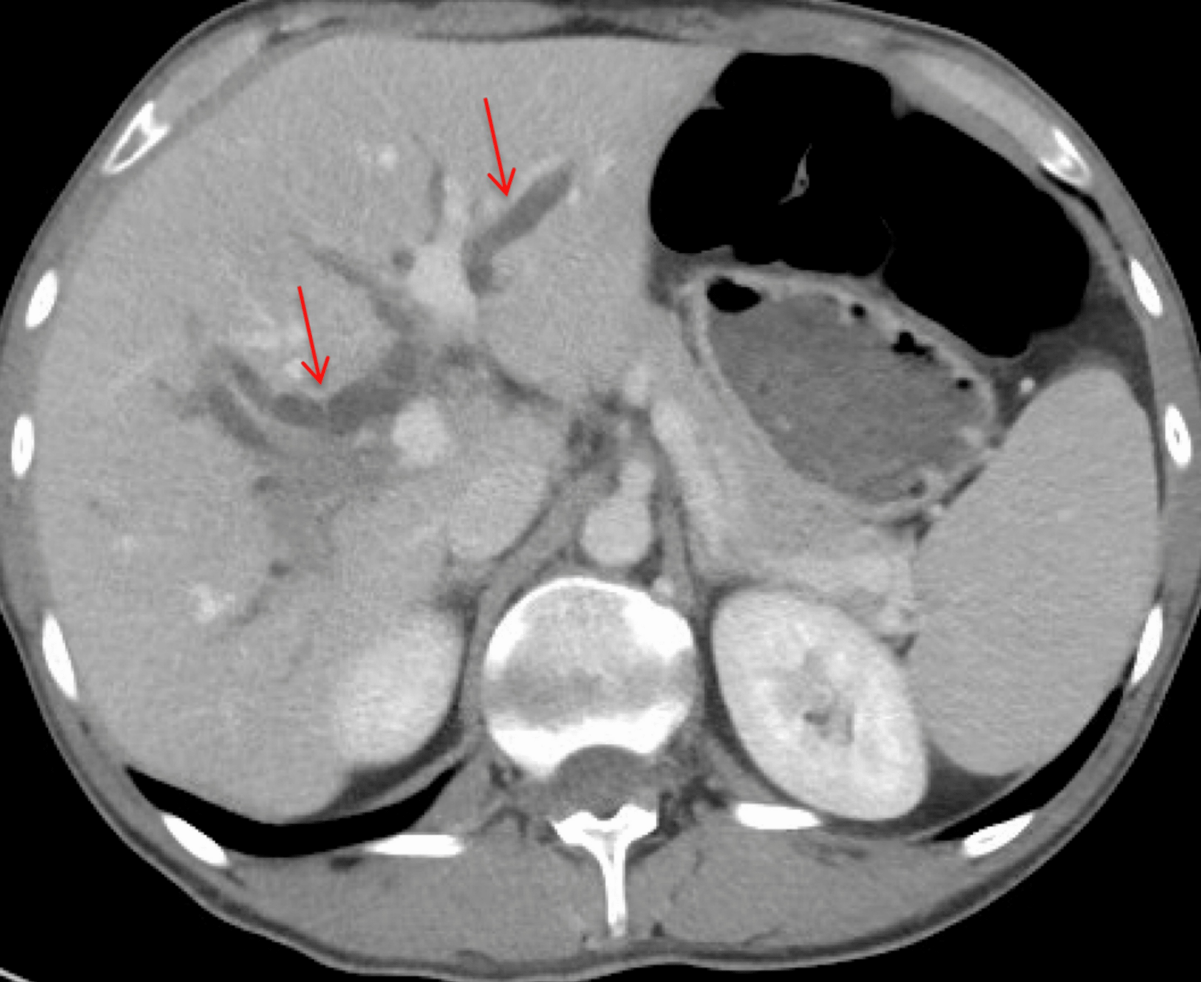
CT abdomen and pelvis with intravenous contrast (axial view) demonstrating intrahepatic and extrahepatic biliary ductal dilation (red arrows) with the CBD measuring up to 11 mm. CBD: common bile duct
